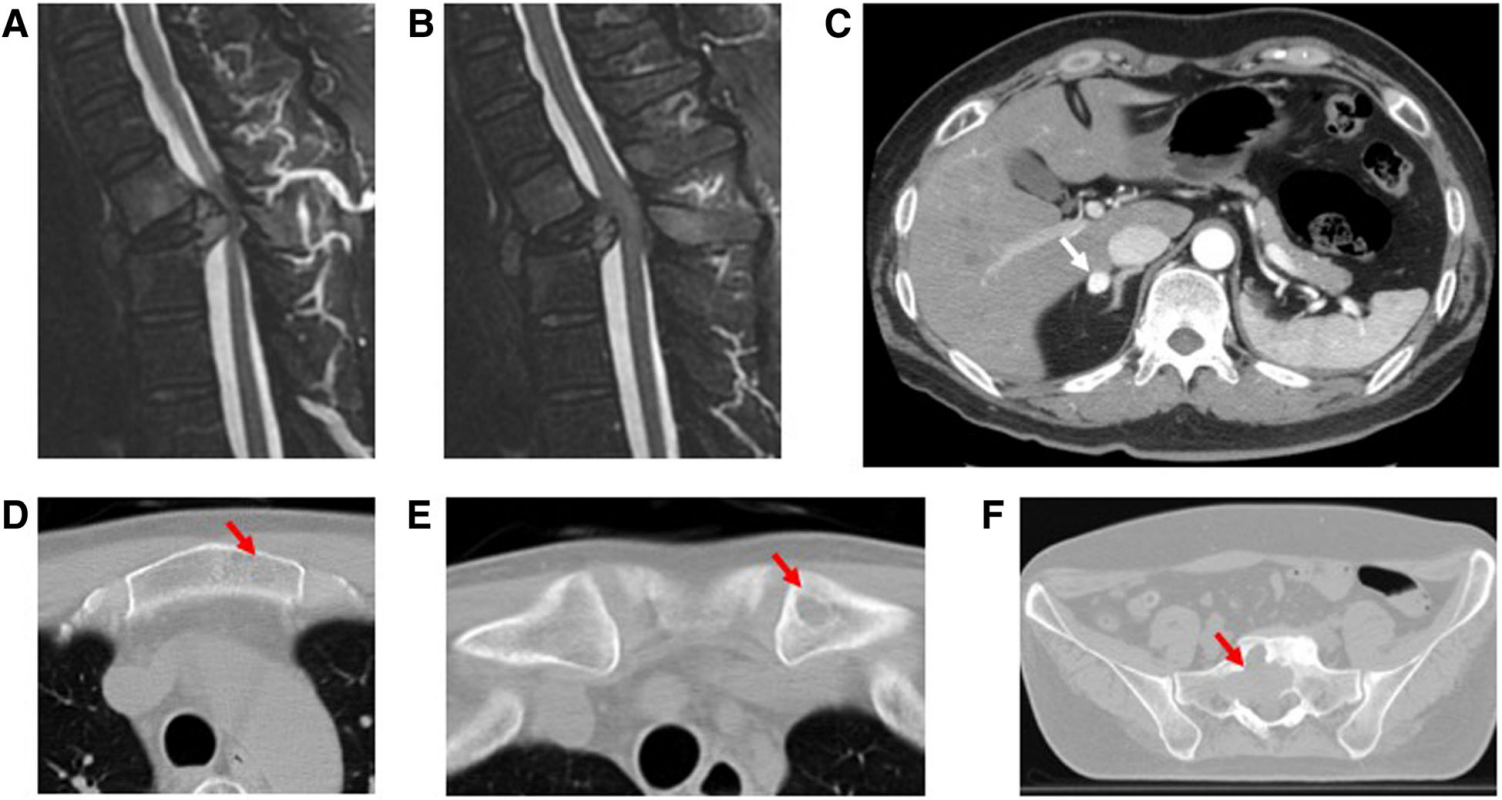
Sagittal magnetic resonance imaging scans demonstrate involvement of the T1–T3 vertebral bodies as well as T2 vertebral collapse (a) and tumor extension causing cord compression at the level of T2 (b). Axial computed tomographic images demonstrate metastases of the right adrenal gland (c), sternum (d), left clavicle (e), and sacrum (f) (*arrows*)
